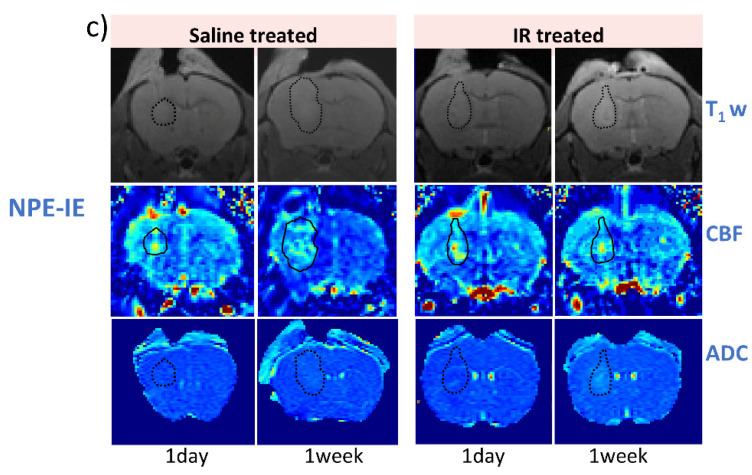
Longitudinal evaluation of tumour response to saline– and IR treatment through MR imaging. Representative T1-weighted, CBF, and ADC mapping of (a) GL261, (b) CT-2A, and (c) NPE-IE models. Segmentation of tumours is indicated by the contoured dotted lines. Darker contrasts represent hypoperfused tumours in the CBF maps, and restricted diffusion in the ADC maps. Brighter contrast represents higher perfusion values in the CBF maps, and less restricted diffusion and/or oedema in the ADC maps.
